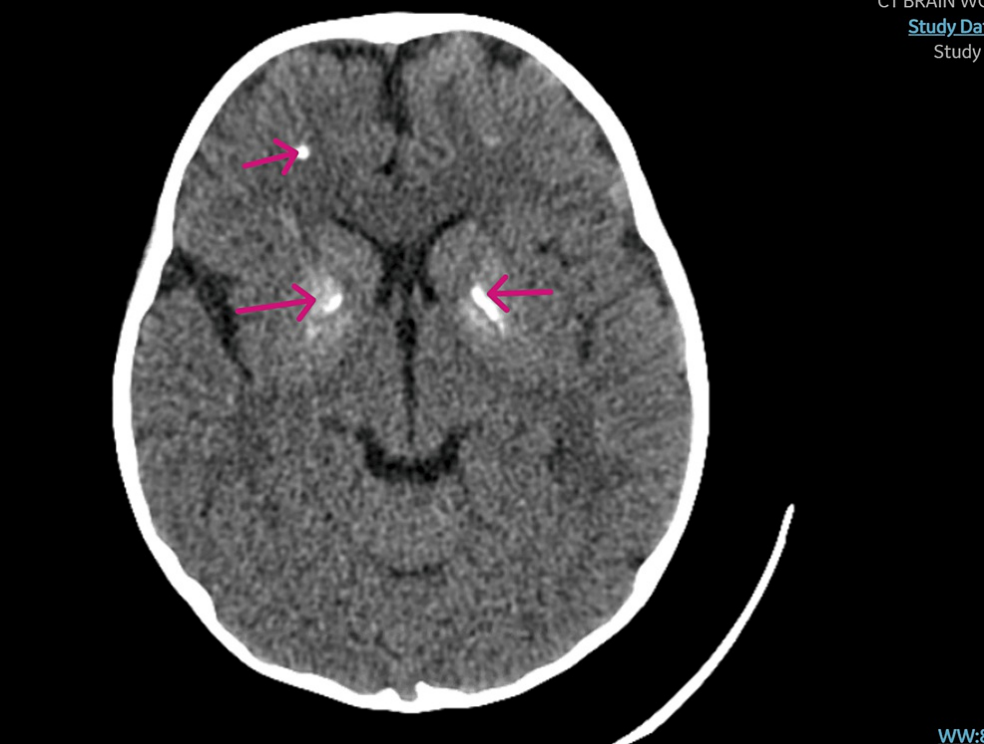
CT scan of the brain, horizontal view No acute well-established territorial infarction or acute intracranial hemorrhage, no mass effect or brain herniation, but multiple calcifications are noted within the basal ganglia, subcortical mainly at the frontal lobe with appropriate size and configuration of the ventricular system and CSF spaces. Posterior structures are grossly unremarkable with the imaging displaying the impression of no acute brain insult. CT: computed tomography; CSF: cerebrospinal fluid
